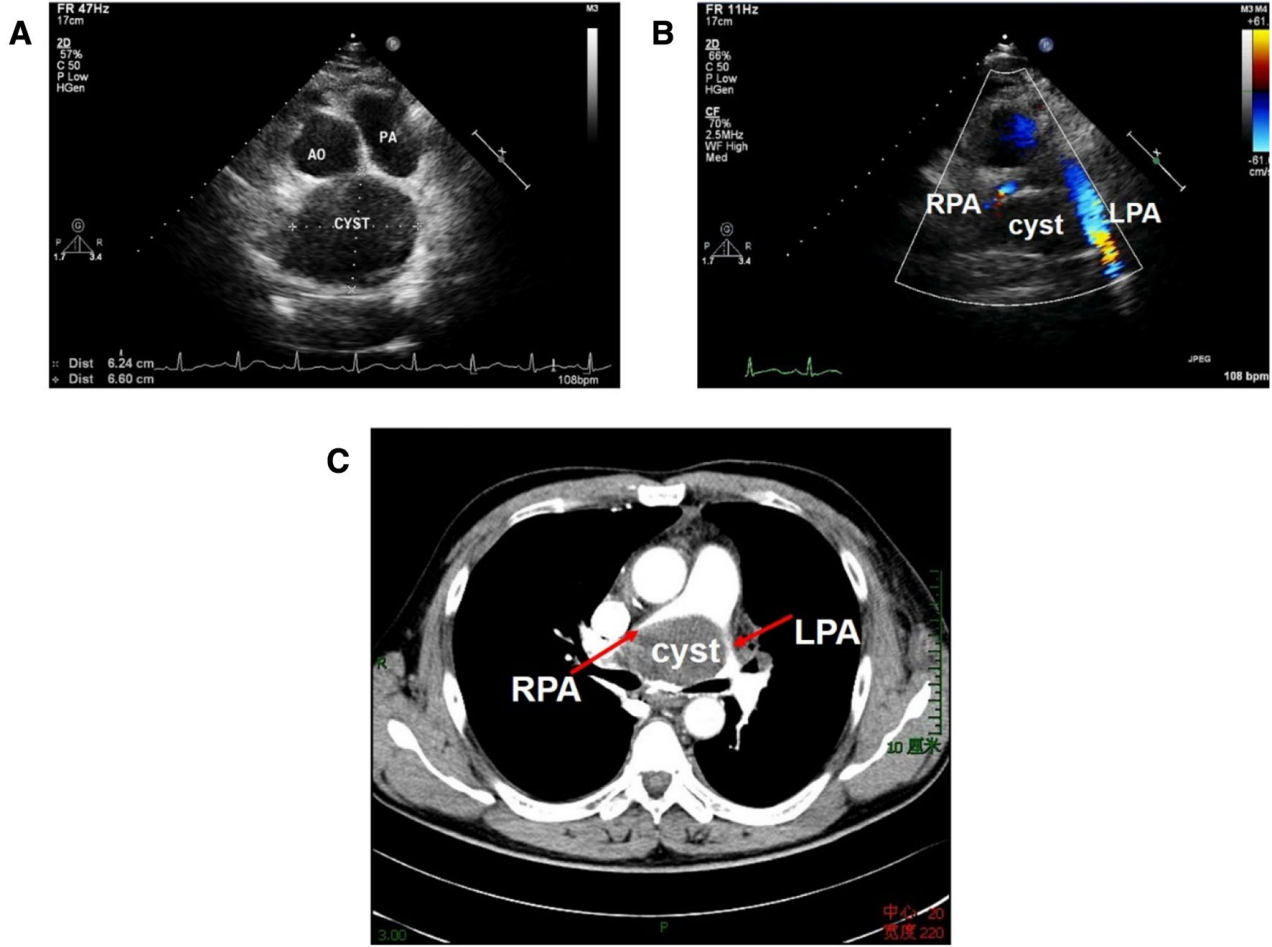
A 47-year-old man with a bronchogenic cyst, complaining of dyspnea and chest pain. a Nonstandard parasternal short-axis view of great arteries showed an echo-free structure in the upper region to the heart; b CDFI demonstrated accelerated blood flows in pulmonary branches resulting from the compression on bifurcation of the pulmonary artery; c Contrast-enhanced CT demonstrated the compression of the cyst. *PA* pulmonary artery, *LPA* left pulmonary artery, *RPA* right pulmonary artery
